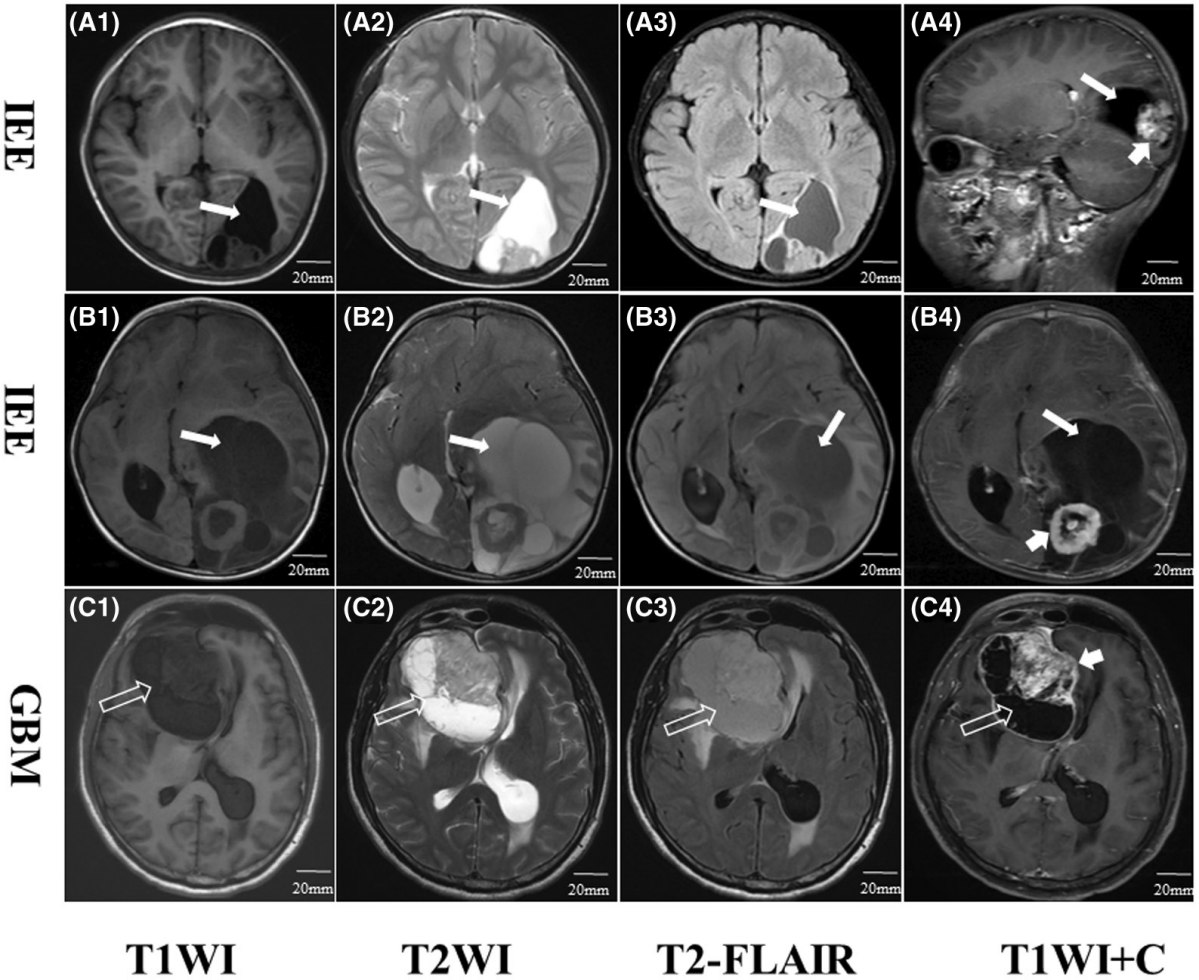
Representative MR images of intracranial extraventricular ependymoma (IEE) and glioblastoma multiforme (GBM). Figures A1–A4: A 3‐year‐old female with IEE (Case 95). Figures B1–B4: A 35‐year‐old female with IEE (Case 100). Figures C1–C4: A 57‐year‐old male with GBM (Case 77). The hollow arrowheads represent tumor necrosis (Figure C1–C4), the long arrows indicate the cystic area within the tumor (Figure A,B). The short arrows represent peripheral enhancing margin (Figure A–C).
